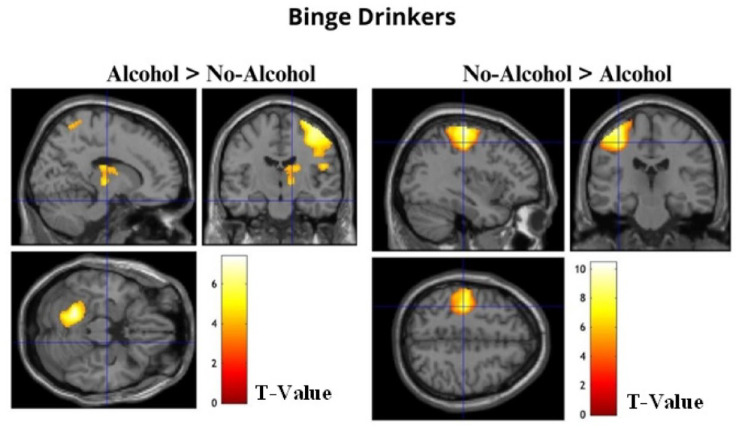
The images show significant activation maps of BDs for the contrasts Alcohol > No-Alcohol and No-Alcohol > Alcohol overlaid on an anatomical MRI image.
